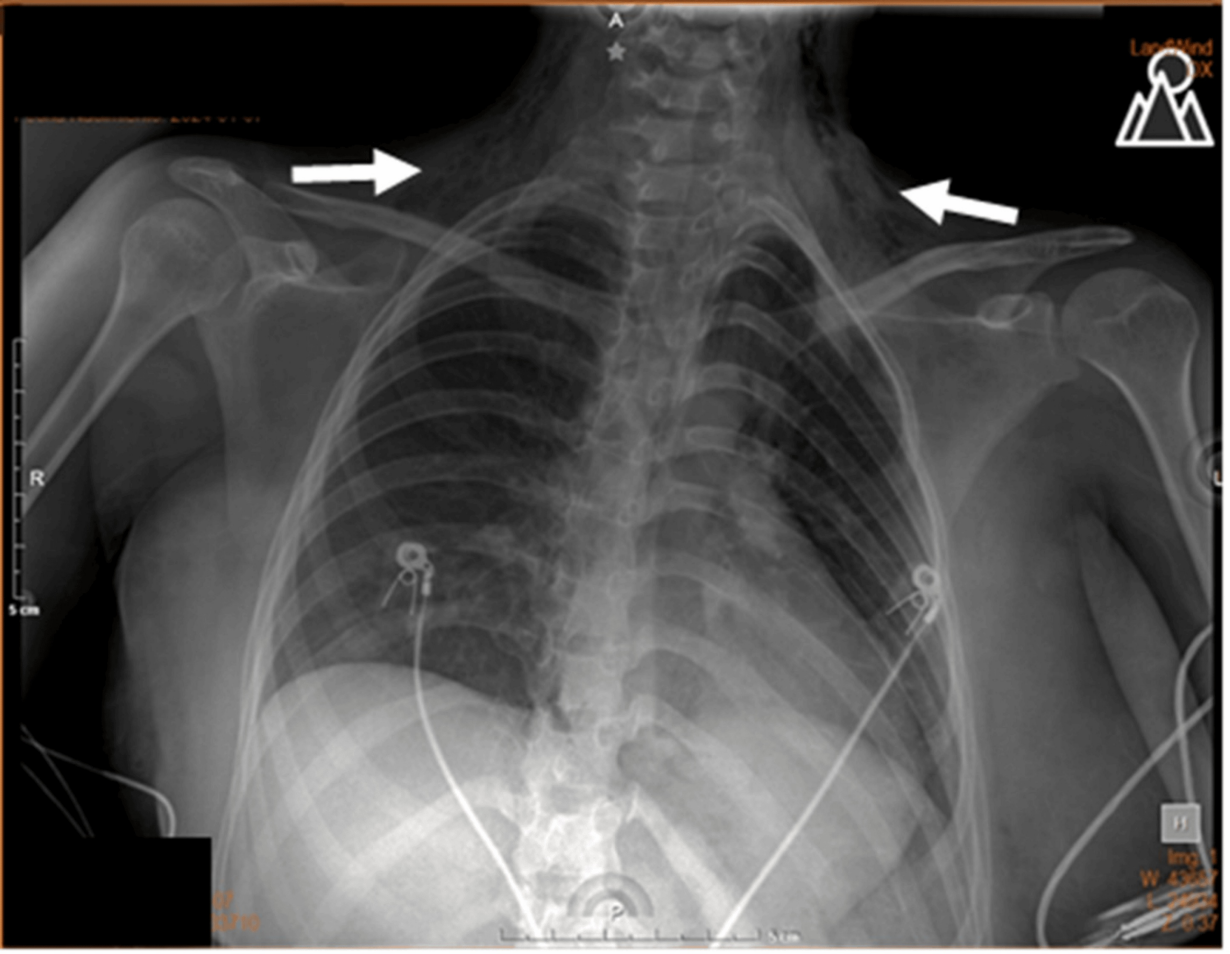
Anteroposterior chest X-ray on day two The image reveals a reduction in the subcutaneous emphysema in the clavicular and cervical regions (white arrows).
